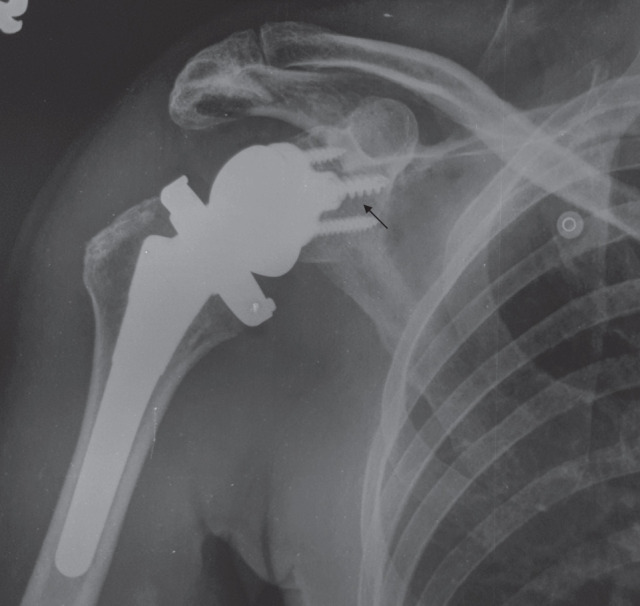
Anteroposterior radiographs showing a shoulder without radiographic signs of loosening after 10 months. No radiolucency is seen around the screws (arrow).
